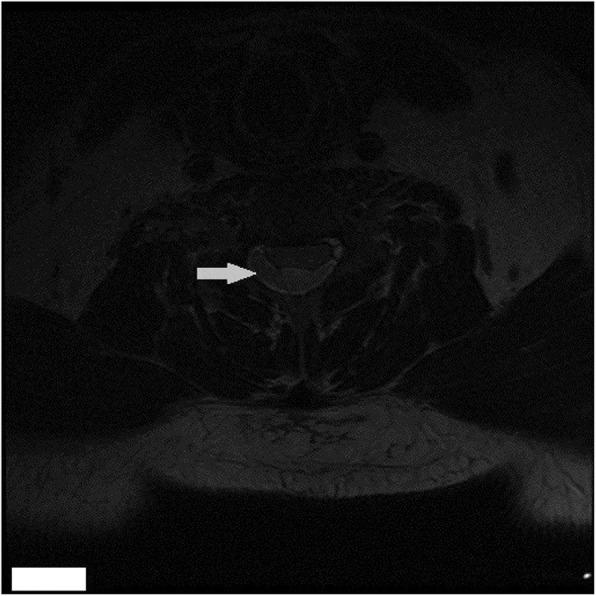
Transaxial MRI of the cervical spine (T2-weighted image). Compression over the right posterolateral aspect of the spinal cord at the C5/C6 level can be seen
